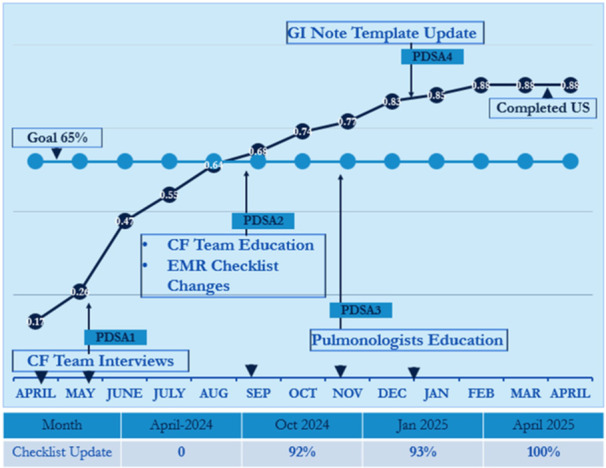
Figure 2: PDSA cycles timeline showing an increase in abdominal ultrasound completion over 9 months. EMR checklists were updated for all PwCF at our center.
